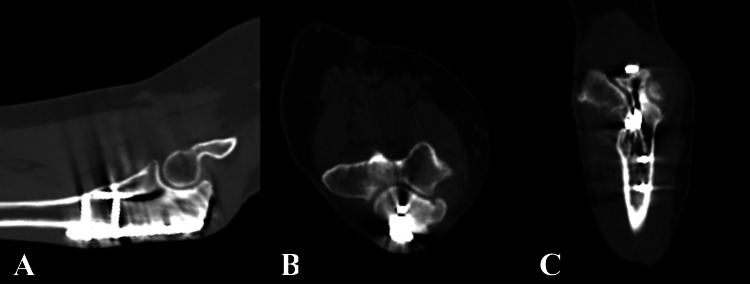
Computed tomography scan of the left elbow. Sagittal (A), axial (B), and coronal (C) slices confirm olecranon fracture union with no residual fracture lines.
